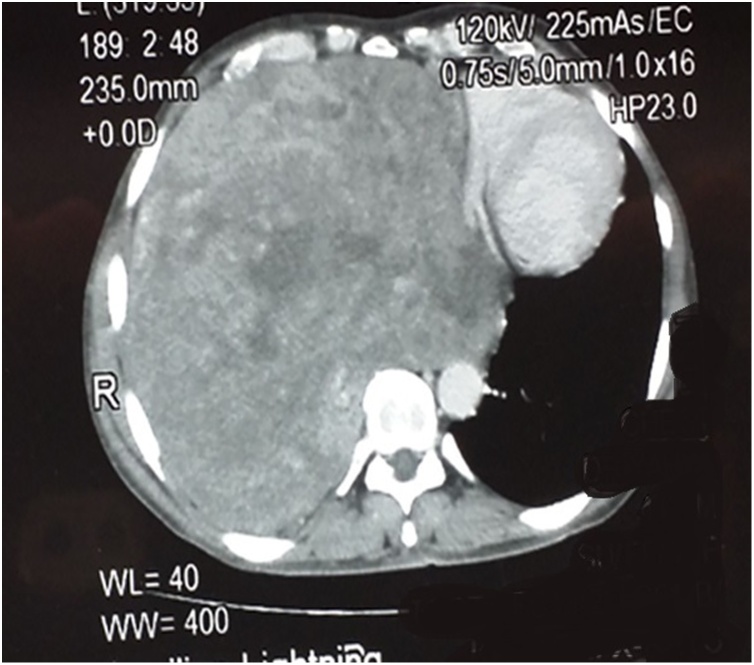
Showing CT imaging of chest demonstrating the mass.
